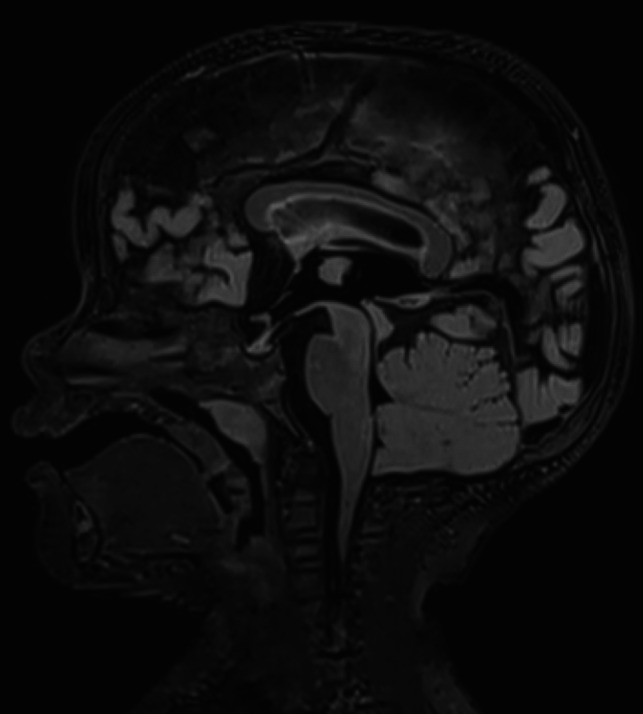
MRI taken 2 years after MMC repair still shows full regression of radiological signs of CM2
